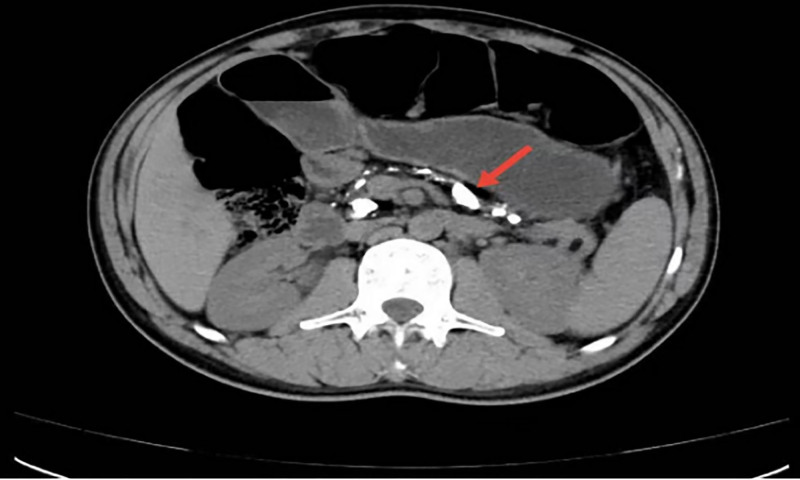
Plain computed tomography abdomen showed multiple calcifications involving the head, body and tail of pancreatic parenchyma.The dilated main pancreatic duct was also seen. (A) Axial plane. (B) Coronal plane.
